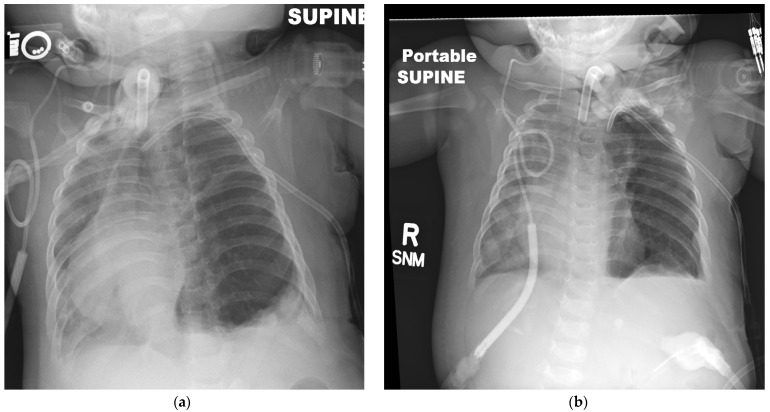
Radiographic findings in the infant. Chest X-ray appearance in the 6-month-old infant status-post repair of CDH, with a tracheostomy and a Broviac^TM^ catheter placement with significant emphysematous left lung with mediastinal shift (a). Conventional therapy with various ventilator modalities and dexamethasone did not result in any improvement (b).
